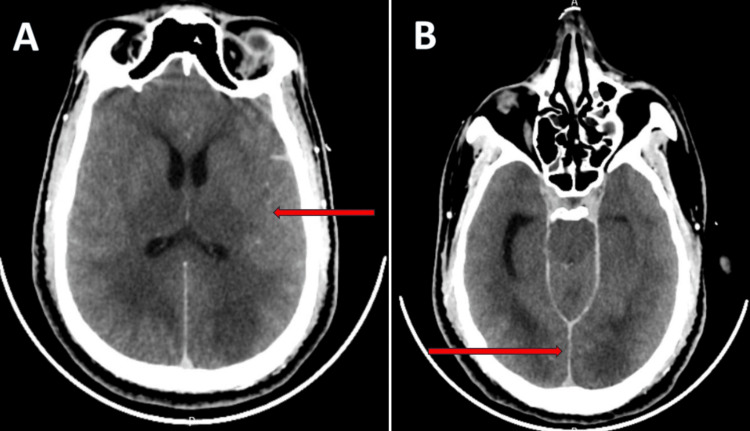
Non-contrast CT brain demonstrating diffuse cerebral edema and subarachnoid hemorrhage (A) Axial CT image shows diffuse cerebral edema with loss of gray-white matter differentiation and effacement of cortical sulci (arrow). (B) Axial CT image demonstrates hyperdensity within the interhemispheric fissure consistent with subarachnoid hemorrhage (arrow). CT: computed tomography
